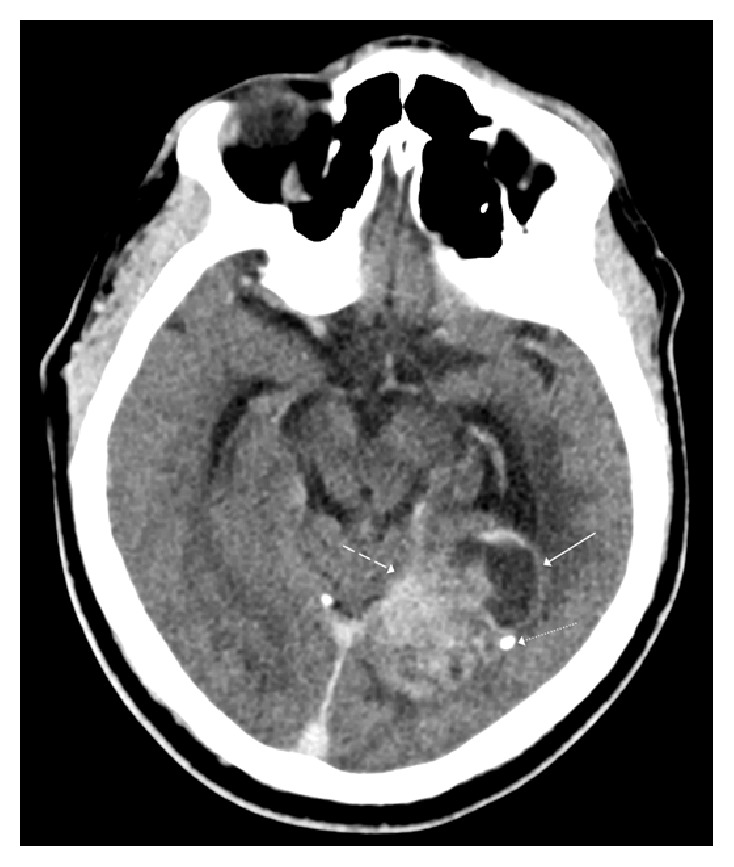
Noncontrast axial computed tomography image shows a mixed solid (dashed white arrow) and cystic (solid white arrow) temporooccipital mass with a hyperattenuating solid component and a punctate calcification peripherally (dotted white arrow).
